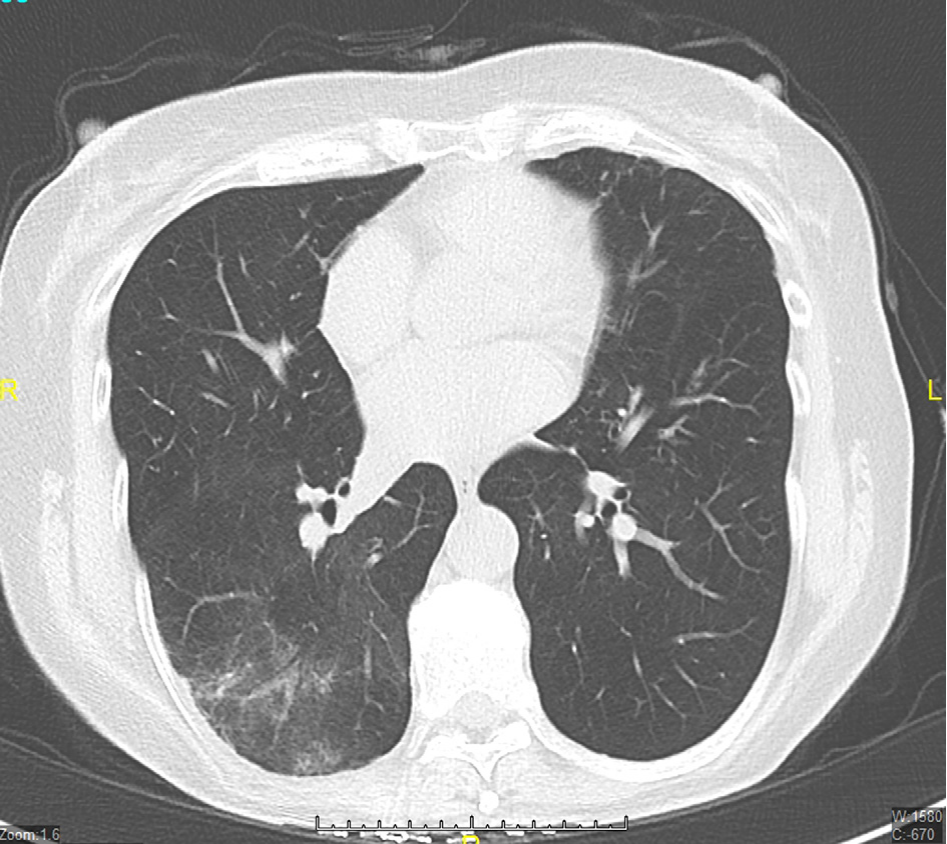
Follow-up CT scan cross section showing near-complete resolution of the consolidation. The test was carried out 8 weeks later.
